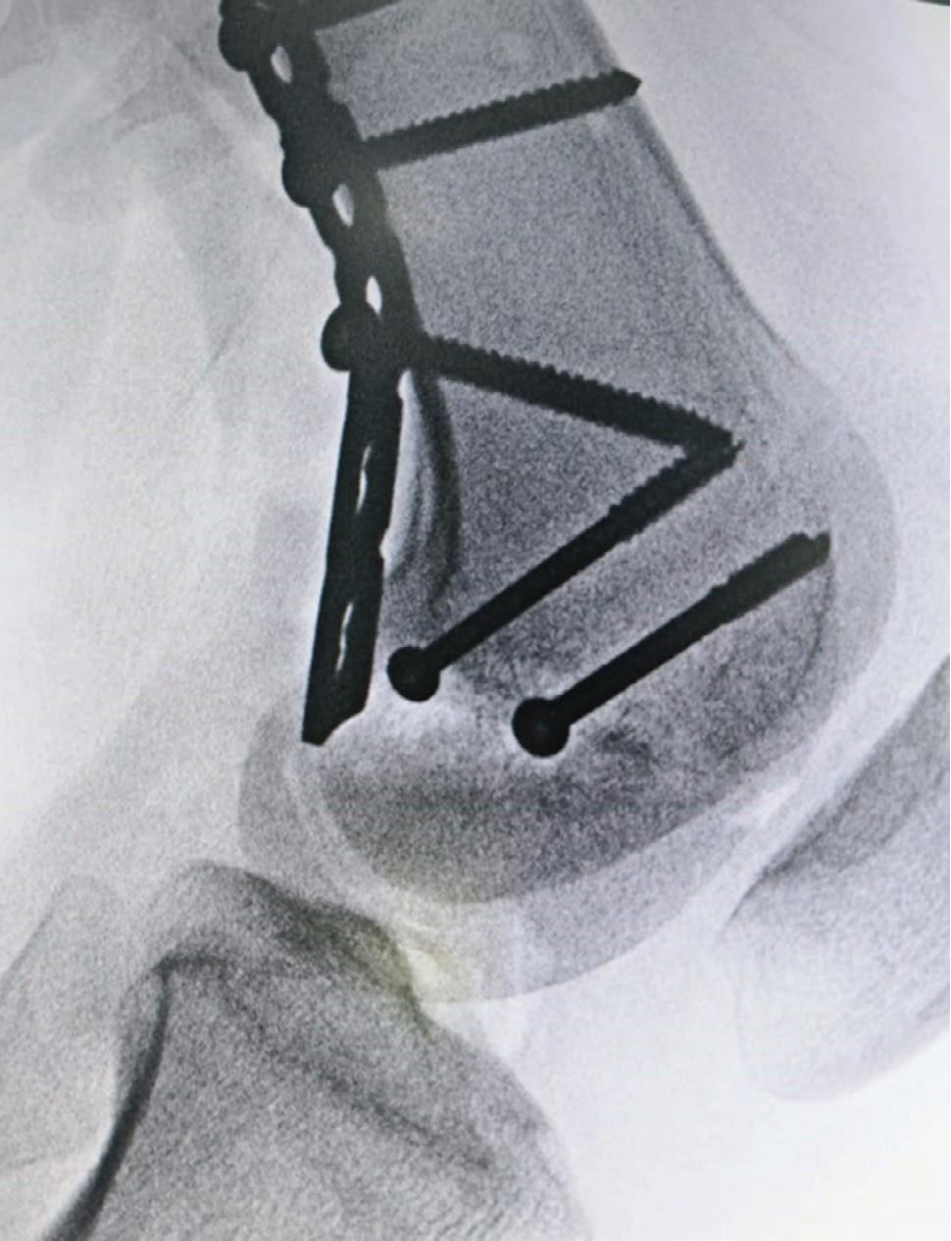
Intraoperative anteroposterior (AP) and lateral radiographs of the right distal femur demonstrating persistent fracture displacement after insertion of two cannulated screws. Image is oriented with anterior structures to the right.
